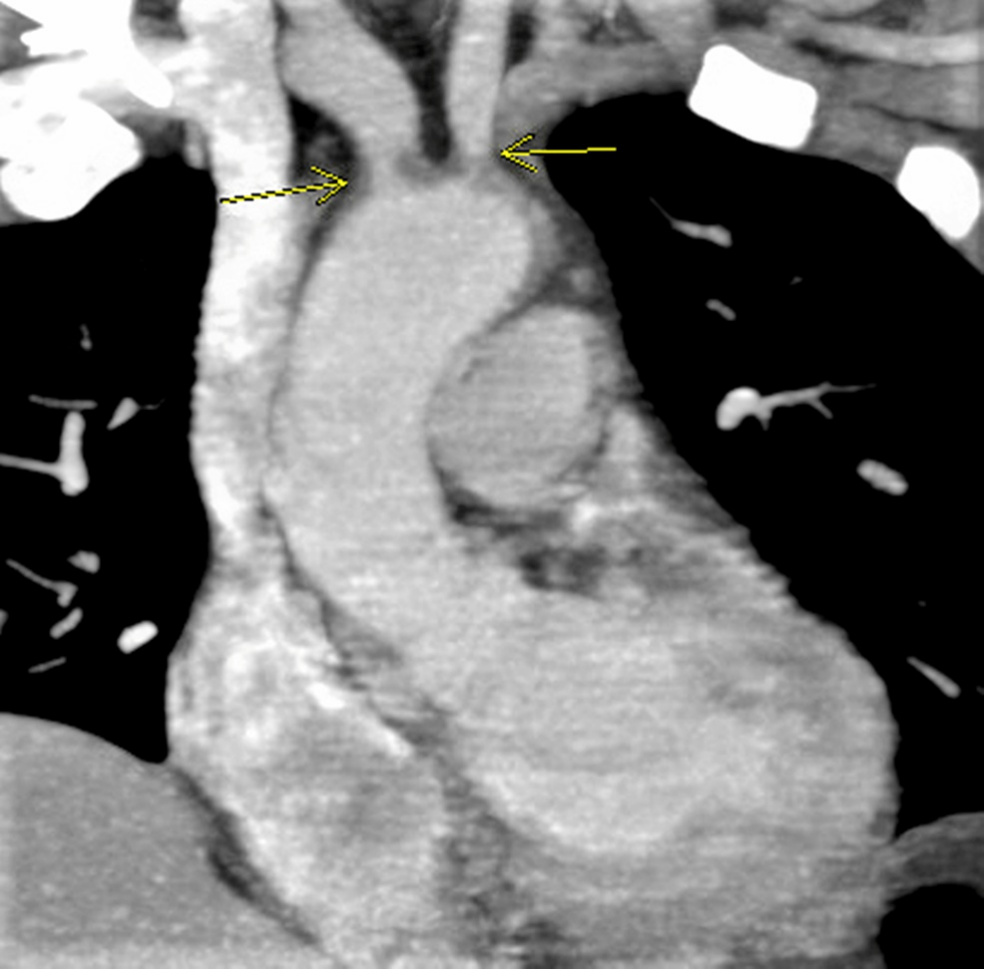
Computed tomography angiography of the neck
